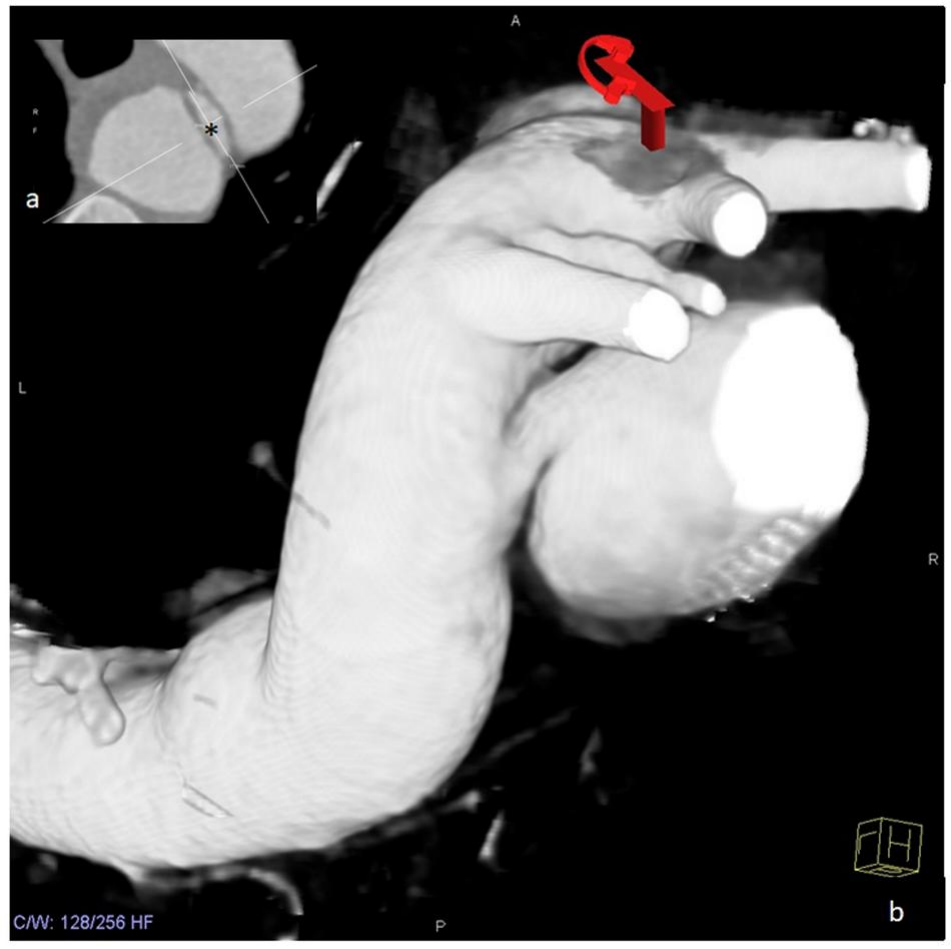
Multidetector CT (3D reconstruction) findings identified the ARSA aneurysm. Inset: an asterisk marks the proximal entrance of the aneurysm with a wide-open base.
